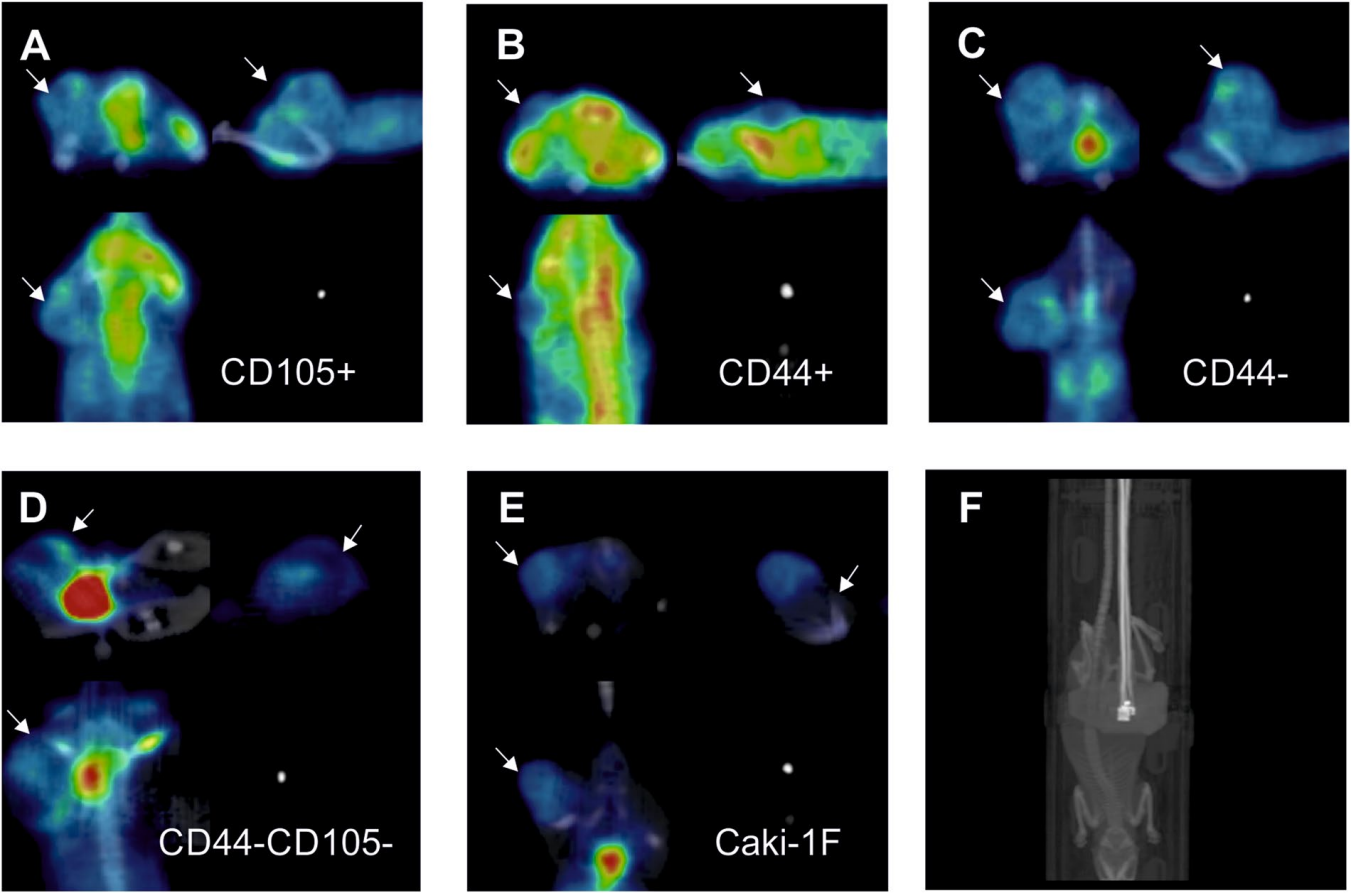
Representative PET images after intravenous administration of 18FDG. Various populations of Caki1F cells: CD105+ (A), CD44+ (B), CD44−, CD44−/CD105− (D) or the unsorted Caki-1F cells (E). The last panel (F) shows a representative CT scan. Arrows point localization of the tumor.
